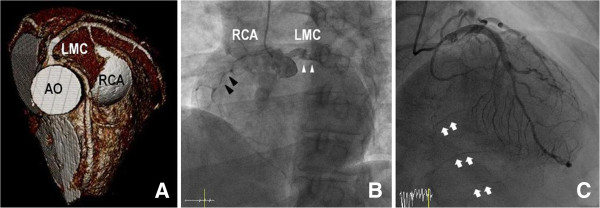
Pre-operative images. (A) Three dimensional volume rendering of contrast-enhanced CT scan shows the anomalous RCA shared the same sinus with the left coronary system, with its attenuated proximal portion passing between the aorta and pulmonary trunk in patient 1. The left coronary system is patent. (B) Coronary angiogram shows anomalous origin of the right coronary artery (black arrowheads) from left sinus of Valsalva, next to the left (white arrowheads) coronary arteries in patient 2. Also it is noted that severe stenotic lesions are present on the RCA. (C) Coronary angiogram in right anterior oblique view shows collateral circulation to the distal RCA (white arrows) via septal branches of the left anterior descending artery, with the normal left coronary artery system in patient 3. (CT: computed tomography; AO: aorta; LMC: left main coronary artery; PA: pulmonary artery; RCA: right coronary artery).
